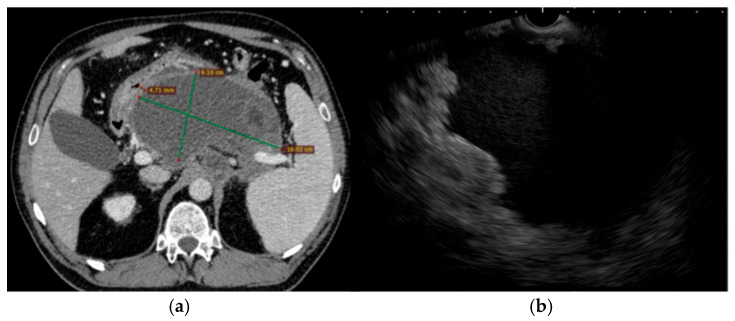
Pre-intervention imaging evaluation. (a). Abdominal CT scan showing a large peripancreatic retrogastric WON (with approximately 30% necrosis) with no parietal pseudoaneurysms, but with marked gastric and mesenteric compression; (b). Trans-gastric EUS image of the same collection of fluid.
